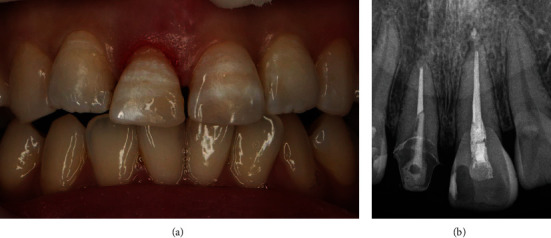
(a) Intra-oral photograph of orthodontic extrusion finished followed by gingivectomy. (b) Periapical view after orthodontic extrusion finished and temporary crown delivery.
